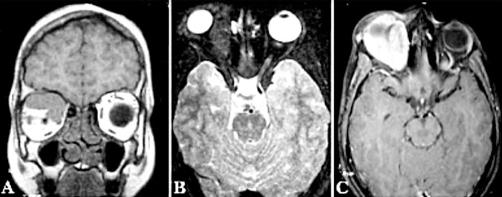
MRI (A) T1 Coronal image; an isointense mass located superio-medially in the right orbit, pushing the orbital contents. (B) T2 axial fat suppressed image; a hypointense, extraconal mass located medially in the right orbit, causing proptosis. No infiltration in the surrounding structures can be seen (C) Post-gadolinium axial image; intense contrast enhancement of the mass.
